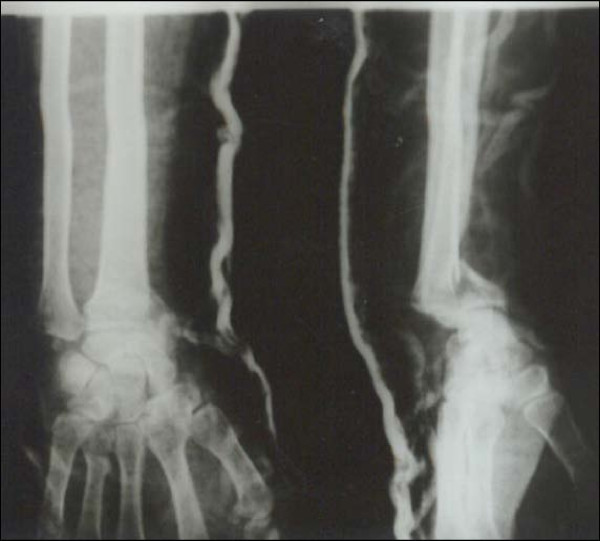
Case 1 Preoperative Radiograph - Anteroposterior and Lateral view.
